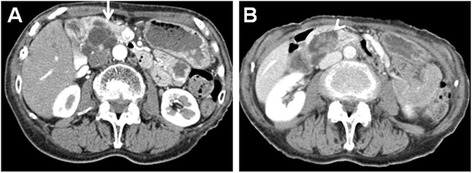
Enhanced computed tomography. Computed tomography showed a cystic lesion with a diameter of 50 mm (A) (arrow) and an irregular mural nodule, which showed gradual enhancement on enhanced CT (B) (arrow head).
